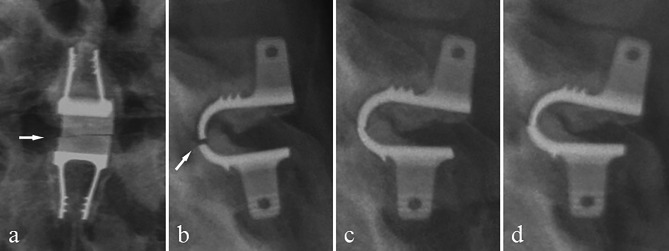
Radiographs of one patient, postoperative image 14 years after surgery. Arrows indicates the fracture
